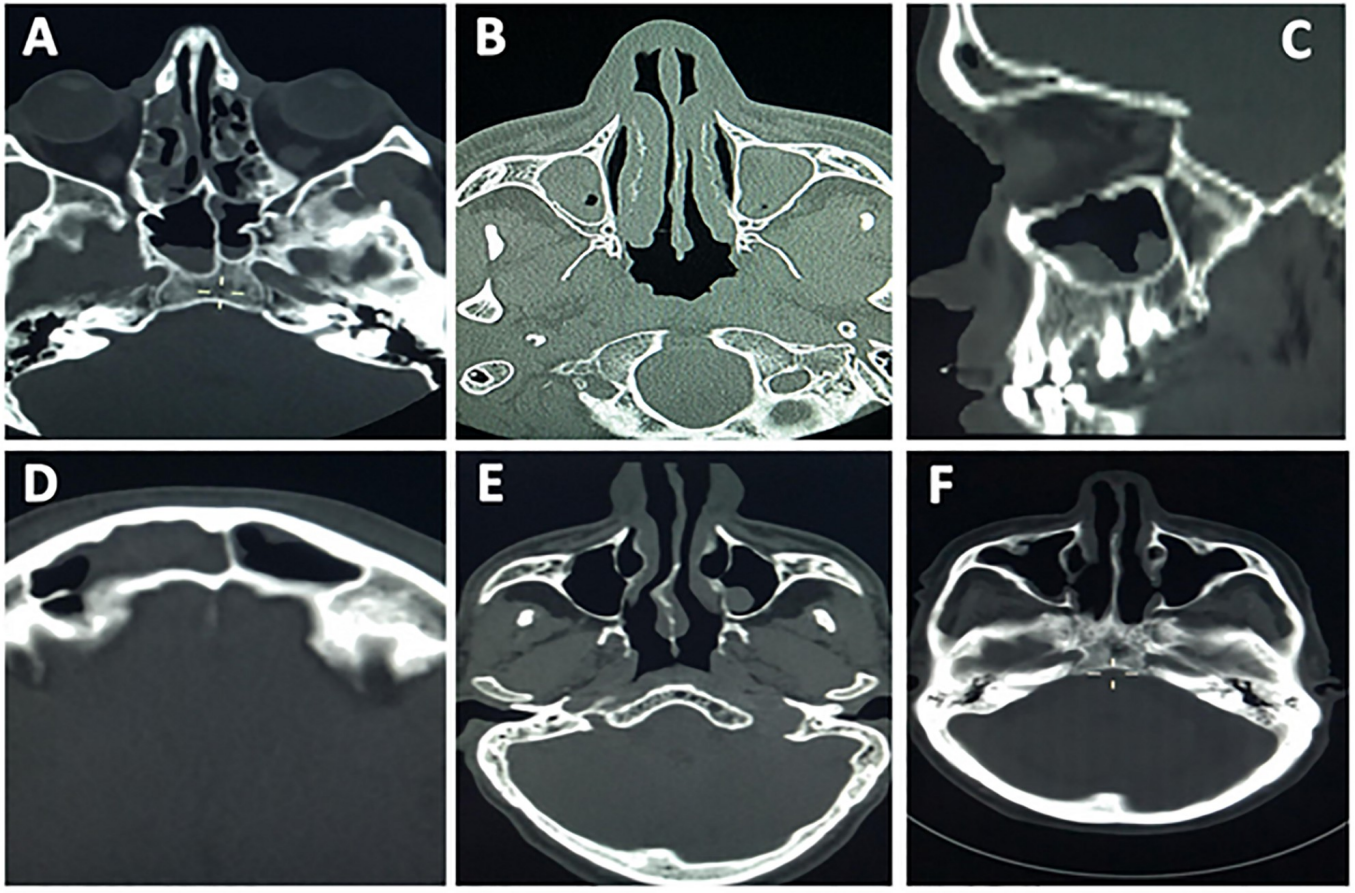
Patients brain CT scans show the incidental detection of paranasal sinuses abnormalities; (A) right maxillary antrum polyp, (B) bilateral maxillary polyp with retention cyst, (C) maxillary antrum polyp and right nasal air fluid level likely abscess, (D) right frontal sinusitis, (E) normal paranasal study, (F) right maxillary antrum mucosal thickening.
